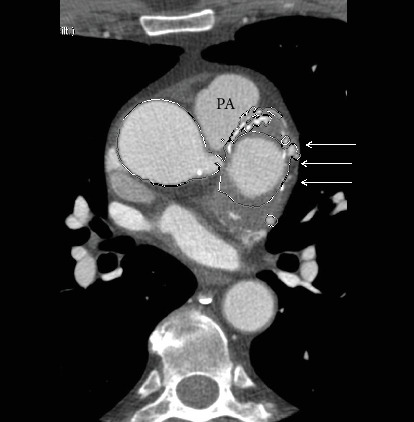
Axial CT image showing the large left SVA causing pulmonary artery (PA) displacement and showing internal calcification and thrombosis (arrows).
